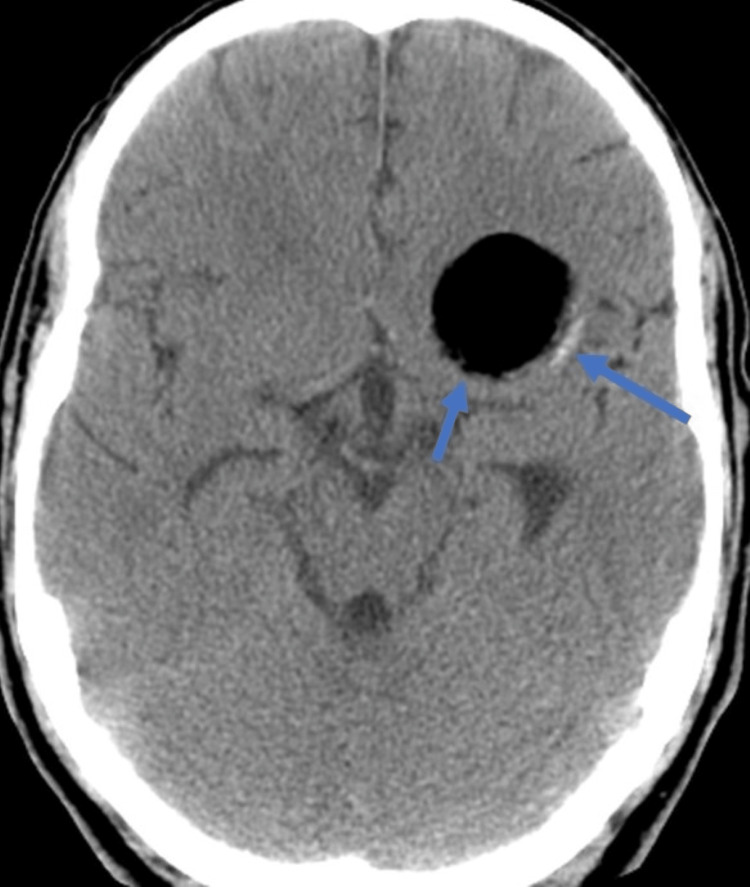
Brain CT revealing a hypodense lesion with smooth margins having cystic component and linear calcifications. CT: computed tomography.
